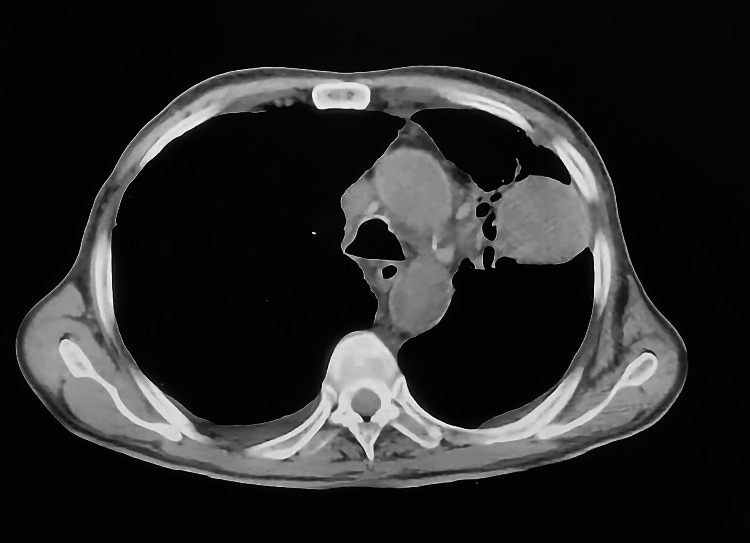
Chest, abdomen, and pelvis CT scan of Patient #2 (2.9× magnification). CT: computerized tomography
